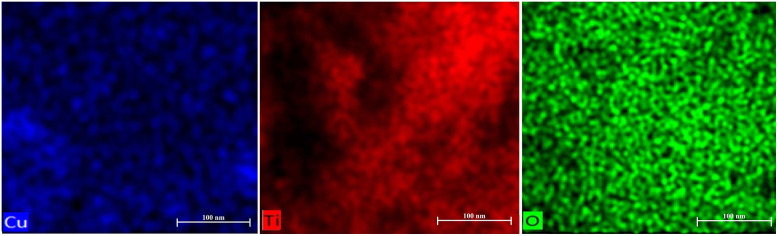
Energy-dispersive X-ray (EDS) elemental mapping images of the synthesized material showing the spatial distribution of Cu, Ti and O. The uniform distribution of Ti and O confirms the formation of a homogeneous TiO_2_ matrix, while the weak Cu signal originates from the copper grid used during SEM/EDS measurements. Scale bar: 100 nm.
